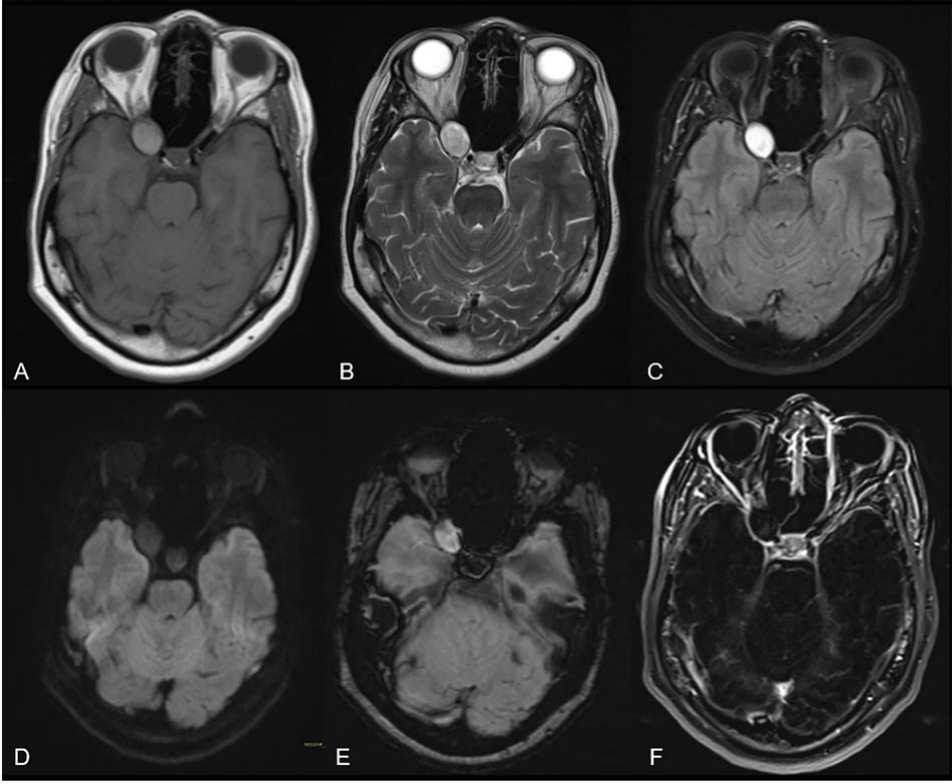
Axial cuts of brain MRI. T1W (A), T2W (B), FLAIR (C), DWI (D), SWI (E) and contrast-enhanced T1W images with subtraction (F); a rounded mass in the right ACP with intrinsic T1 high-intensity signal, suggesting a proteinaceous content with smooth, linear and peripheral mucosal enhancement; no restricted diffusion or susceptibility artifacts were observed.
